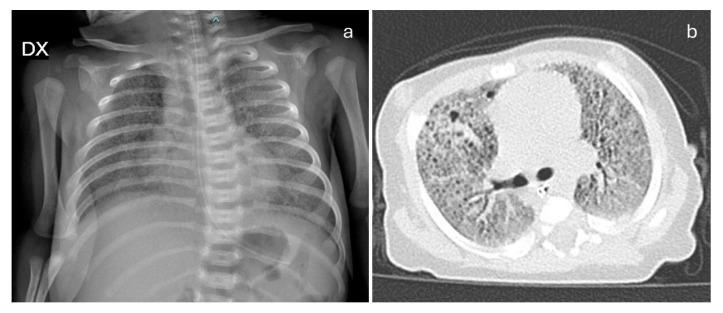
(a) Chest X-ray shows hypodiaphany in all lung areas, in addition to texture reinforcement with a reticulo-micronodular pattern; (b) Chest CT: in both lungs, a diffuse increase in parenchymal density is observed, with a predominantly “ground glass” appearance and associated thickening of the inter- and intralobular septa. Bilateral, multiple, subcentimeter-sized air-filled cavities are present.
